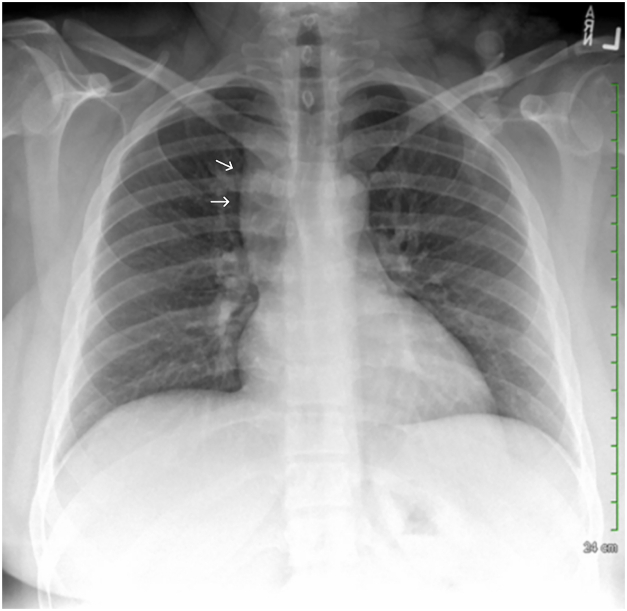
Chest roentgenogram. Posteroanterior view showing a right-sided mediastinal shadow widening at the level of the ascending aorta (arrows).
